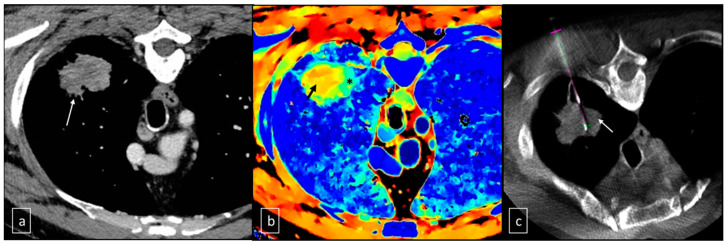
(a) Native axial CT acquisition, performed after intravenous administration of iodized contrast medium, in the venous phase demonstrates the hypodense pulmonary lesion (white arrow). (b) Z-effective axial reconstruction at the same level, which demonstrates reduced cellularity in the central portion of the lesion (color map from yellow to red—black arrow) and greater cellularity at the periphery (color map from green to blue—asterisk). (c) Intra-procedural axial CBCT image that demonstrates correct positioning of the cutting needle for sampling at the most peripheral portion of the lesion, probably with greater tumor cellularity, in the area of max Z value (white arrow). This sequence of images also shows the homogeneous pattern of the lesion tissue after contrast administration, so it was not discriminable the vital neoplastic tissue from the fibrotic one.
